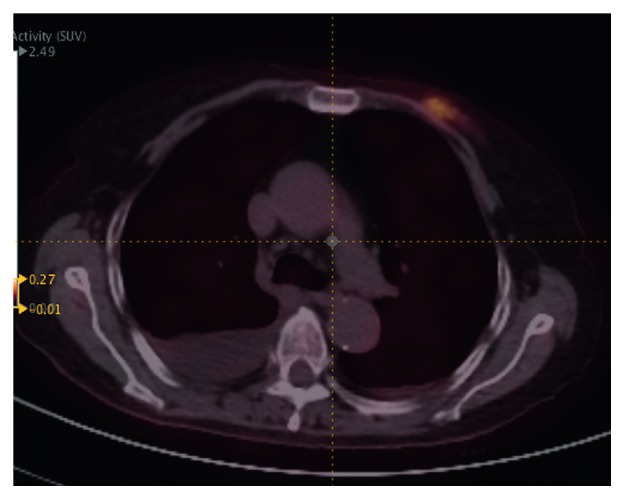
An example of lymphoma that was misdiagnosed as carcinoma by the three radiomic predictive models and the SUV method (SUV_max_ = 2.1, PET radiomic parameters: HISTO_Entropy_log10 = 0.74, SHAPE_volume (# vx) = 34, GLCM_Entropy_log10 = 1.07, GLRLM_HGRE = 17.1, NGLDM_contrast = 0.158, GLZLM_HGZE = 17.5; CT radiomic parameters: HISTO_kurtosis = 21.11. SHAPE_volume (mL) = 2.325, GLCM_homogeneity = 0.382, GLRLM_RLNU = 279.2, NGLDM_busyness = 0.050, GLZLM_LZHGE = 183718.048).
